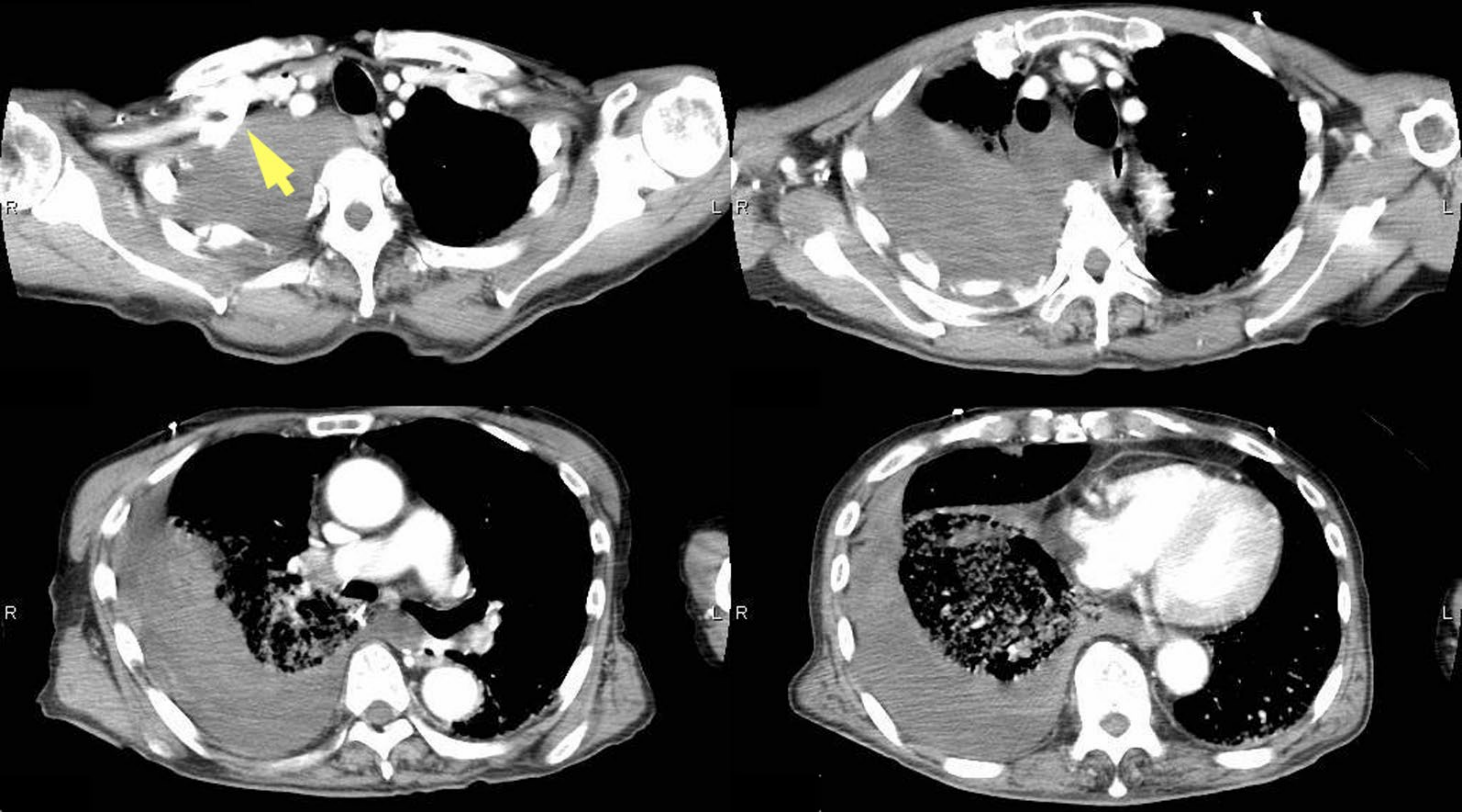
Contrast-enhanced CT. Contrast-enhanced CT reveals massive pleural effusion with high CT attenuation, suggestive of a blood component, along with contrast agent leakage within the pleural cavity. Initially, the source of bleeding was suspected to be the right subclavian artery (yellow arrow)
